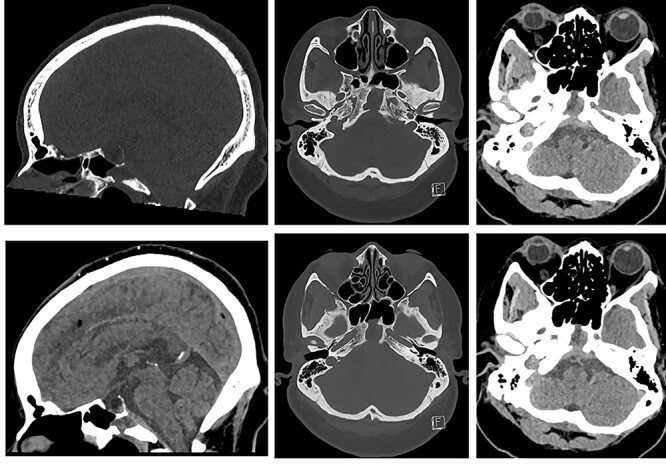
CT of the head (left: sagittal bone and soft tissue windows, and, right: axial bone window and soft tissue window at the same levels ×2) showing sphenoid mucopyocele, retroclival dural thickening and increasing clival dehiscence (anterior and posterior cortex).
